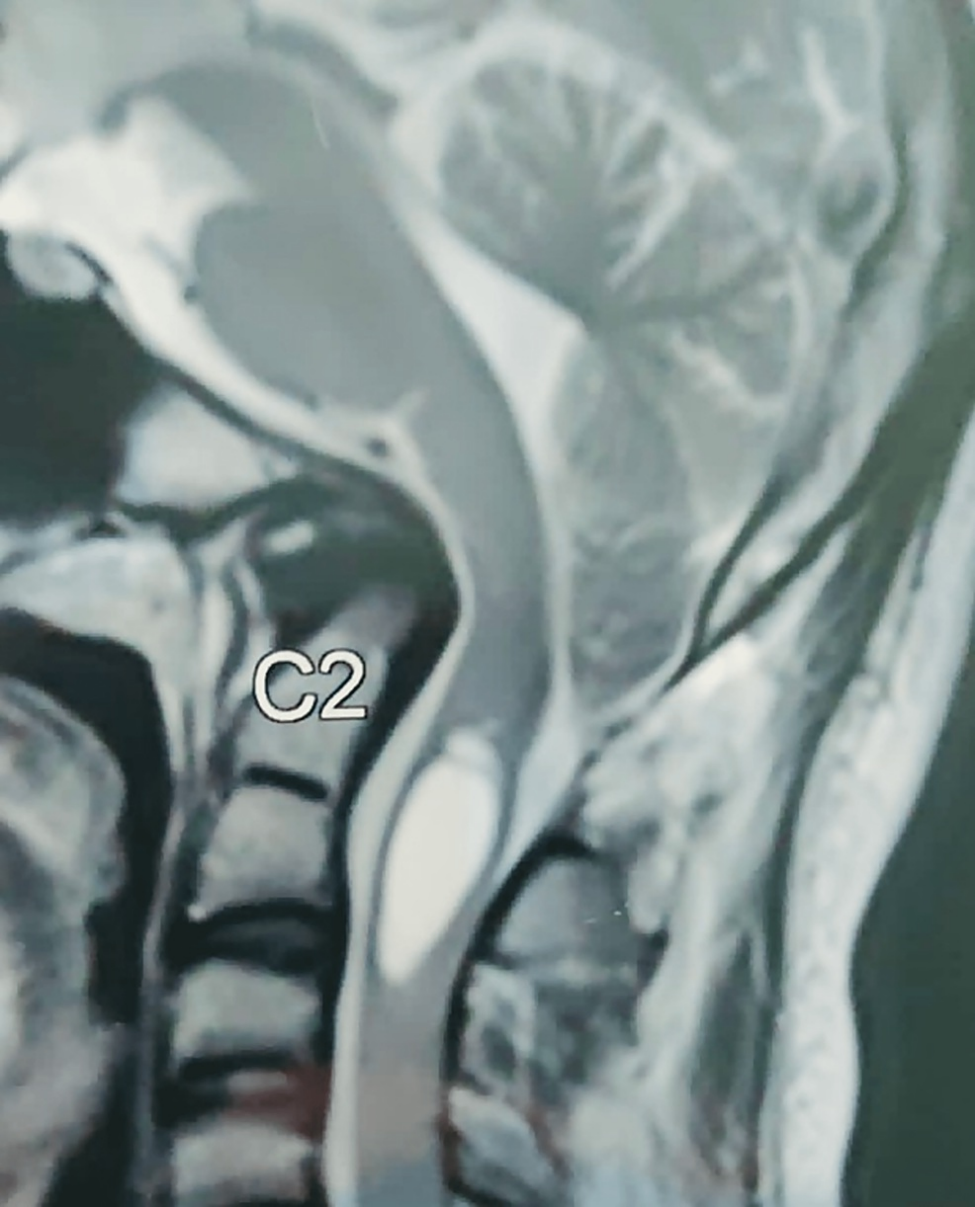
T2-weighted MRI (sagittal) showing cerebellar tonsil herniation through the foramen magnum with an intramedullary syrinx
